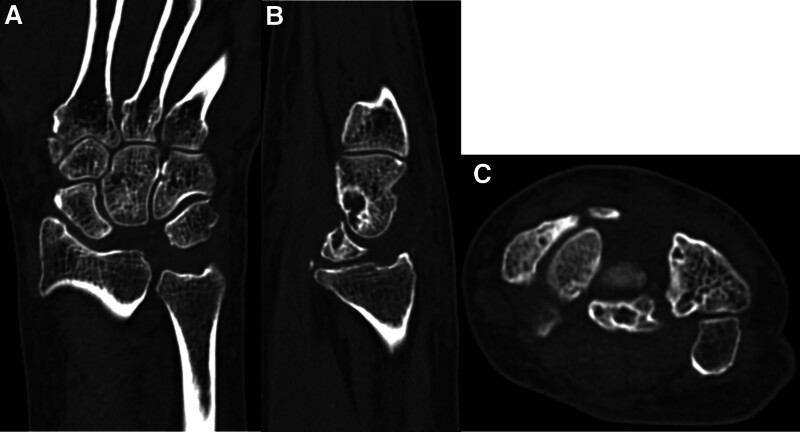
Plain computed tomography at 6 mo after surgery. (A) Coronal, (B) sagittal, and (C) axial view of right wrist indicating the dorsal fragment of the lunate bone was removed, and the volar fragment was retained.
